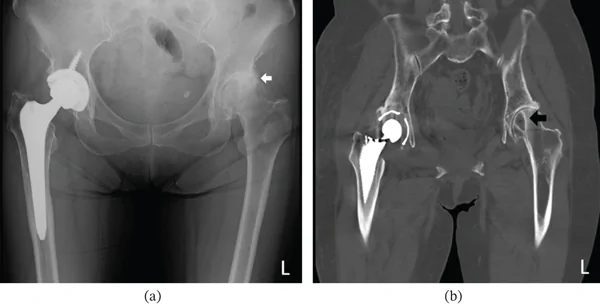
Imaging findings for a 74‐year‐old woman who presented with progressive left thigh pain. (a) Anteroposterior radiograph of the pelvis demonstrating subtle joint space narrowing and osteophyte formation at the femoral head, with no evidence of cortical thickening or beaking in the left femur (white arrow). (b) Coronal computed tomography image showing bone cysts in the left femoral head (black arrow).
